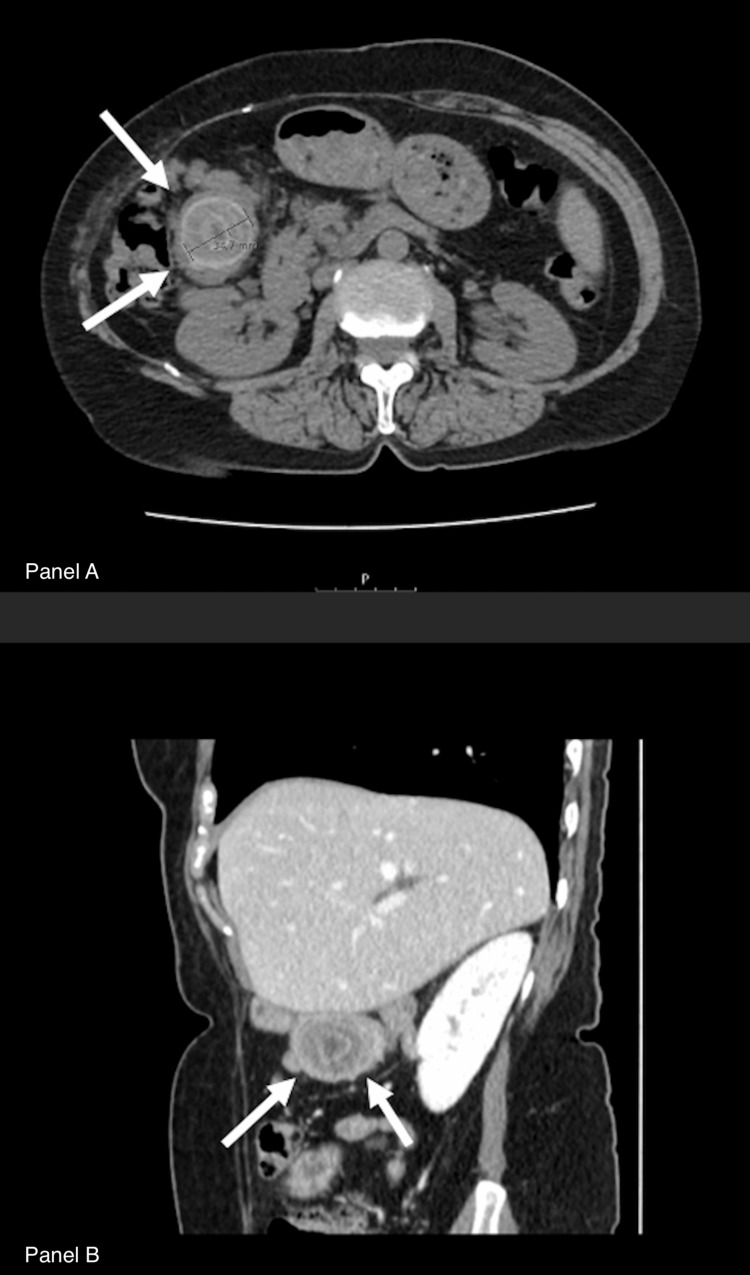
Abdominal CT scan showing the gallstone impacted in the small bowel Panel A: Transverse plane. Arrows showing the biliary stone. Panel B: Coronal plane. Arrows showing the biliary stone.
